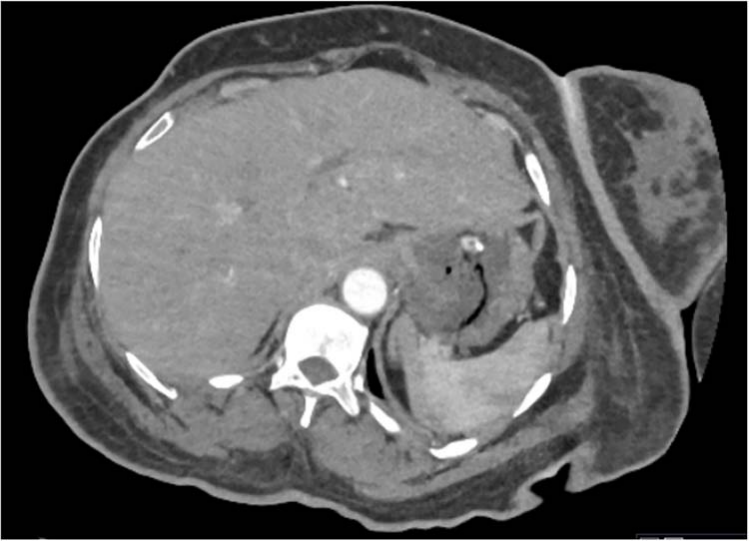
CT abdomen and pelvis image demonstrating interval resolution of portal venous gas, at the level of T11/12.
